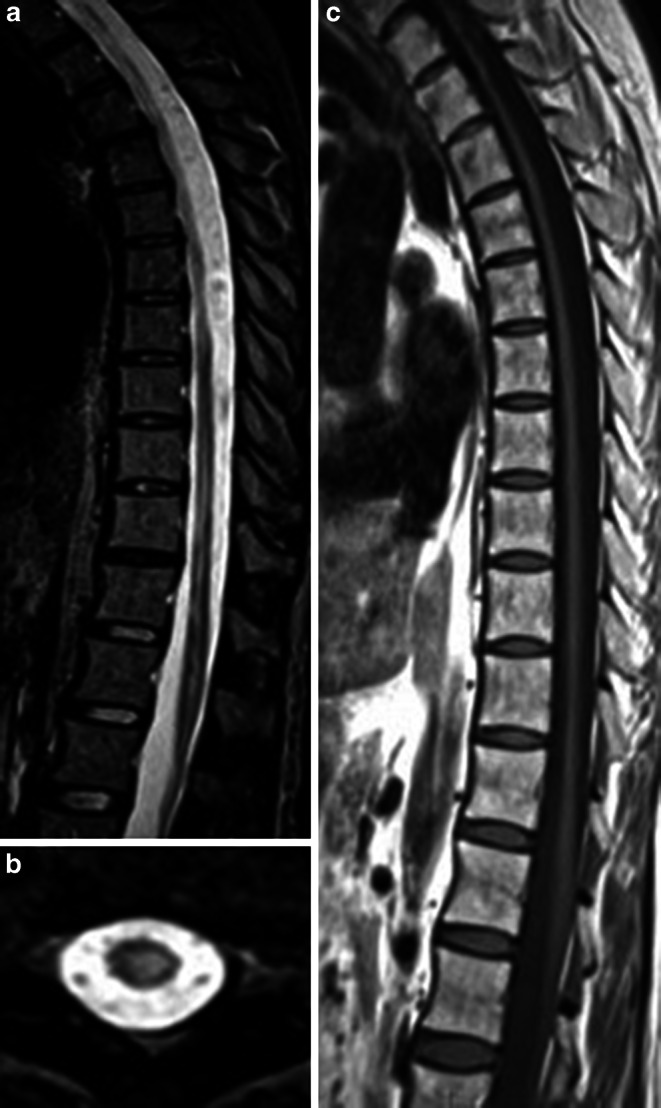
MRI of spinal cord atrophy in a patient with PPMS. Spinal cord atrophy in a patient with PPMS a T2-weighted image in the sagittal plane; marked atrophy of the thoracic spinal cord segments with visible hyperintense signal abnormalities. b T2-weighted image in the axial plane of thoracic segment (Th9–Th10), marked atrophy with hyperintense signal reflects demyelinating lesion. c T1-weighted image, sagittal plane in the same patient with marked atrophy in thoracic spinal cord
